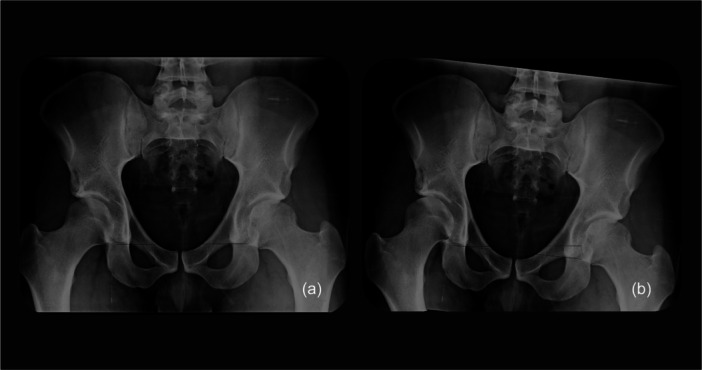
(a) Antero‐posterior (AP) pelvis radiograph. (b) Pelvis radiograph showing the adjusted radiograph with femur and the pelvis rotated according to the peak angles of pelvic drop and femoral adduction extracted from the coronal plane running biokinetic analysis (right hip).
